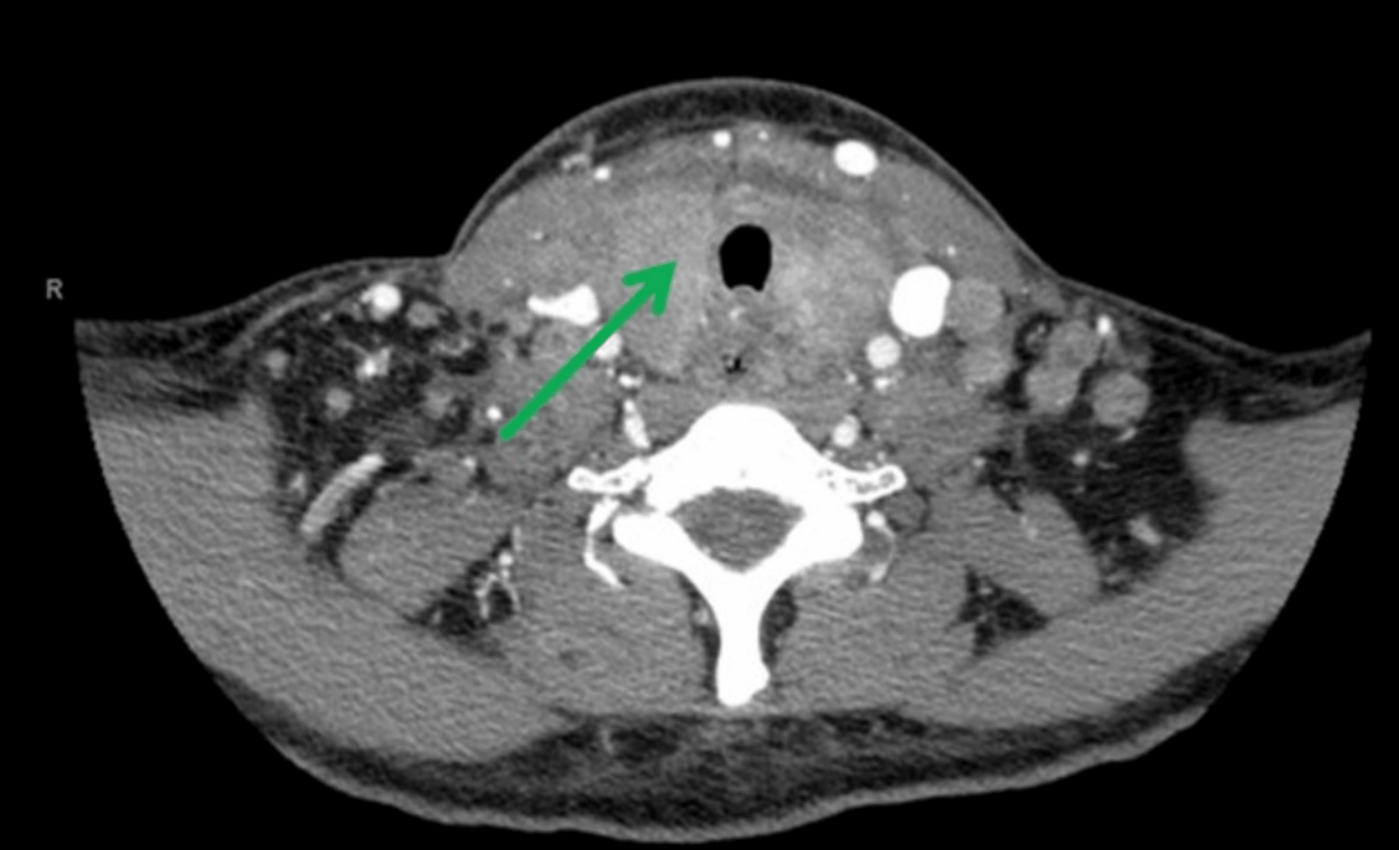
CT neck with contrast demonstrating indistinct thyroid gland (green arrow).
